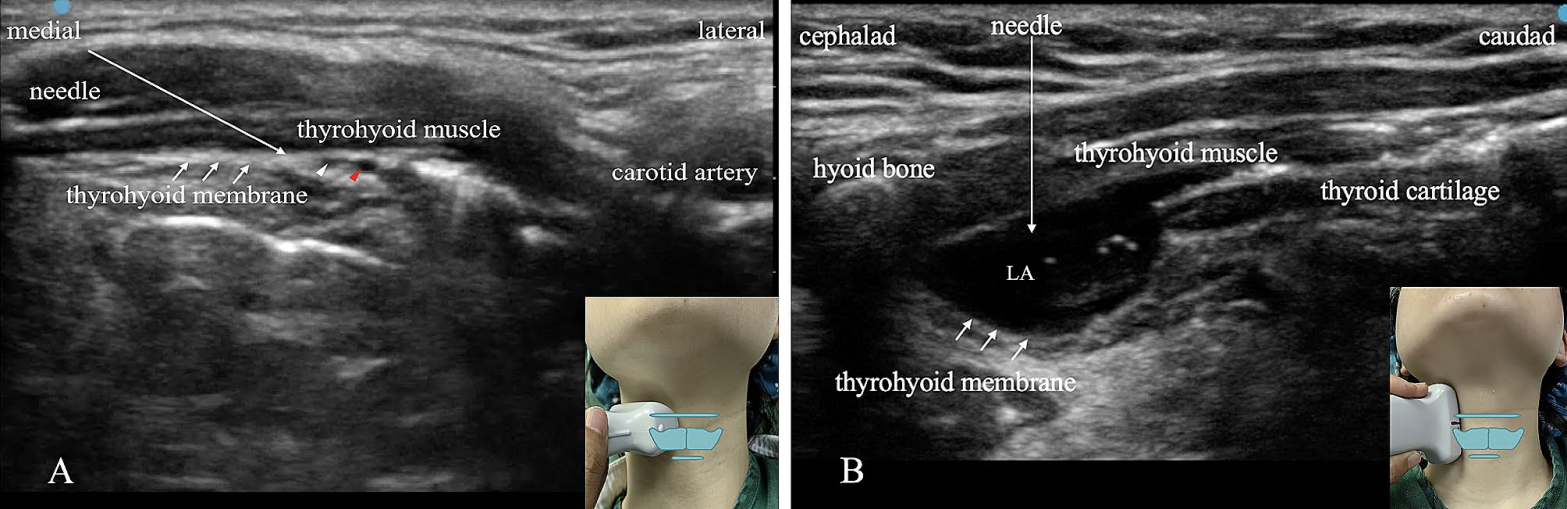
(A), Transverse approach ultrasound-guided SLN block with an in-plane technique. Long arrow: block needle; short arrow: thyrohyoid membrane; white short arrow head: superior laryngeal nerve; red short arrow head: superior laryngeal artery. (B), Parasagittal approach ultrasound-guided SLN block with an out-of-plane technique. Long arrow: block needle; short arrow: thyrohyoid membrane; LA: local anesthetic
